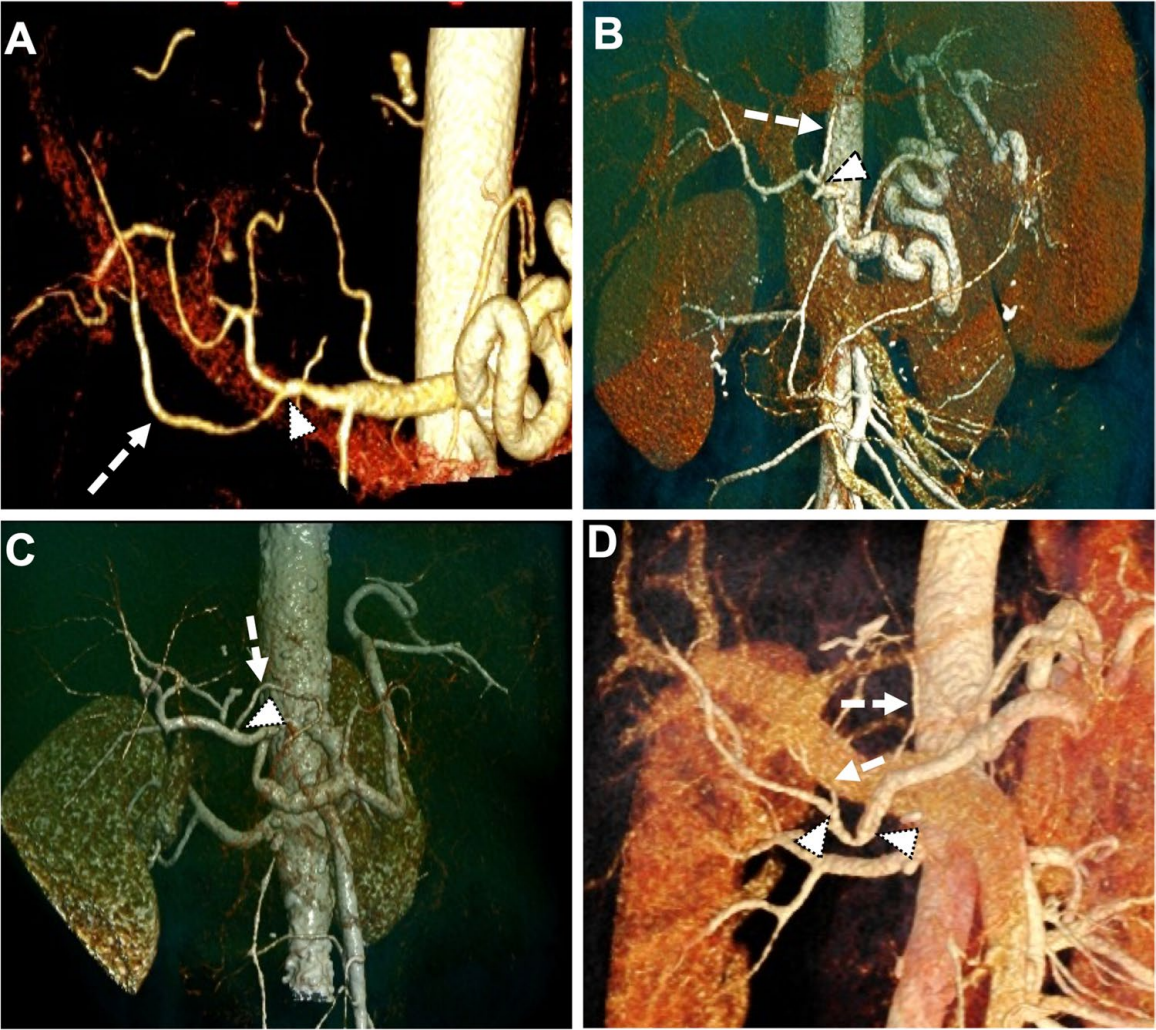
Computed tomography with selective reconstruction of the vascular anatomy. Representative images from 4 scans showing a reconstructed (A) replaced right hepatic artery, (B) replaced left hepatic artery, (C) accessory left hepatic artery, and (D) accessory left and right hepatic artery. White arrowheads with black lining indicate the site of reconstruction, and white arrows indicate the reconstructed vessel
